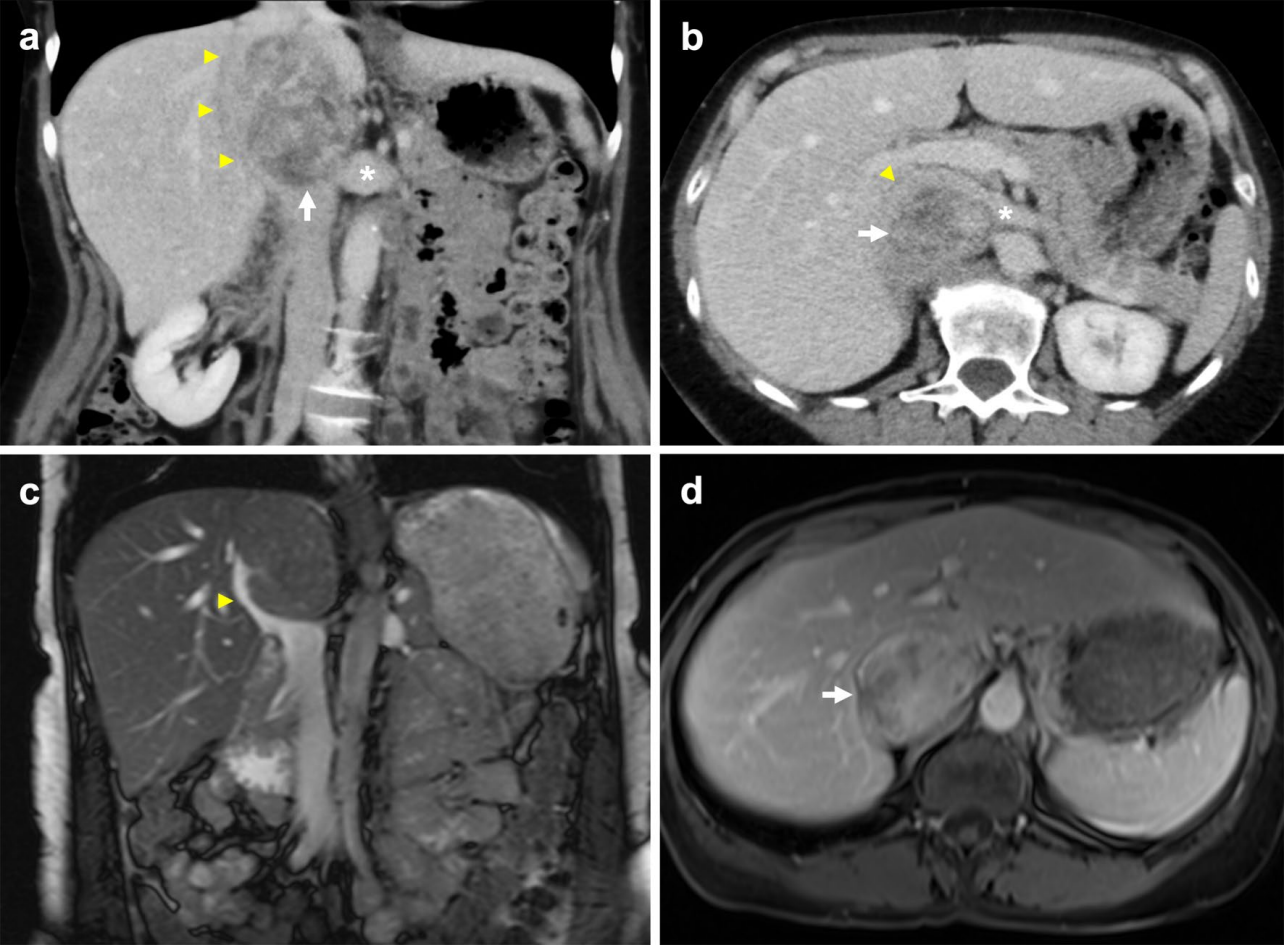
IVC Leiomyosarcoma mistaken for adrenal lesion. a,b Coronal and axial contrast-enhanced CT demonstrate a heterogeneous mass (white arrows) displacing the left renal vein (asterisks) anteriorly and hepatic cava (yellow arrowheads) anterolaterally to the right. c T2-weighted axial MR image shows the effacement of the hepatic cava (arrowhead) without invasion of adjacent structures. d Contrast-enhanced T1-weighted fat-saturated axial image demonstrates heterogeneous enhancement of the tumour (arrow)
